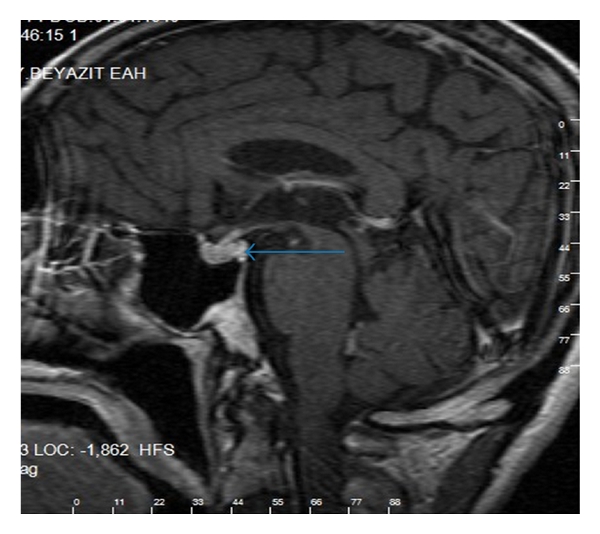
MRI showed that 5 × 8 mm mass the has irregular borderline (arrow).
